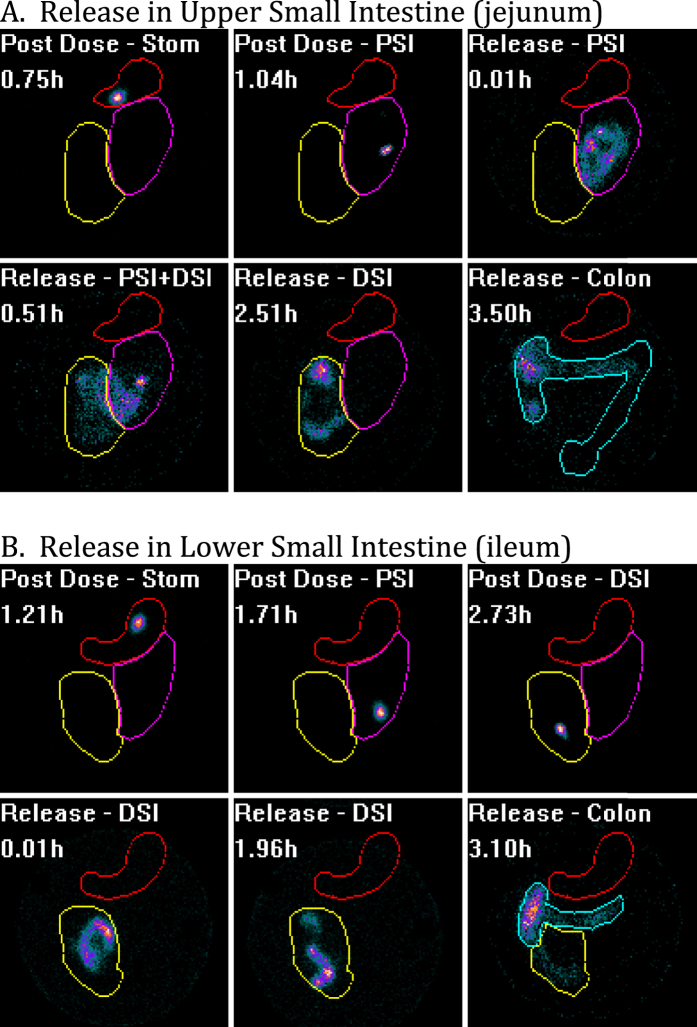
Scintigraphy visualization of subjects given vaccine released in the upper small intestine (A) versus the lower small intestine (B). Each subject swallows vaccine in a size 000 mechanical capsule loaded with liquid vaccine and a radiolabeled tracer. Post dose, one can visualize the capsule transiting through the stomach (Stom, red circle) and the intestine as a discrete spot because the radiolabeled tracer is all contained with the capsule. As the radiolabeled tracer proceeds through the proximal small intestine (PSI, purple circle) and the distal small intestine (DSI, yellow circle), the figures are labeled with such. Post release, the liquid contents are ejected from the capsule and dispersion of the material can be visualized as it spreads away from the capsule. When verified that the dispersed material has reached the colon (Colon, green outlined region), scintigraphy visualization is no longer required.
